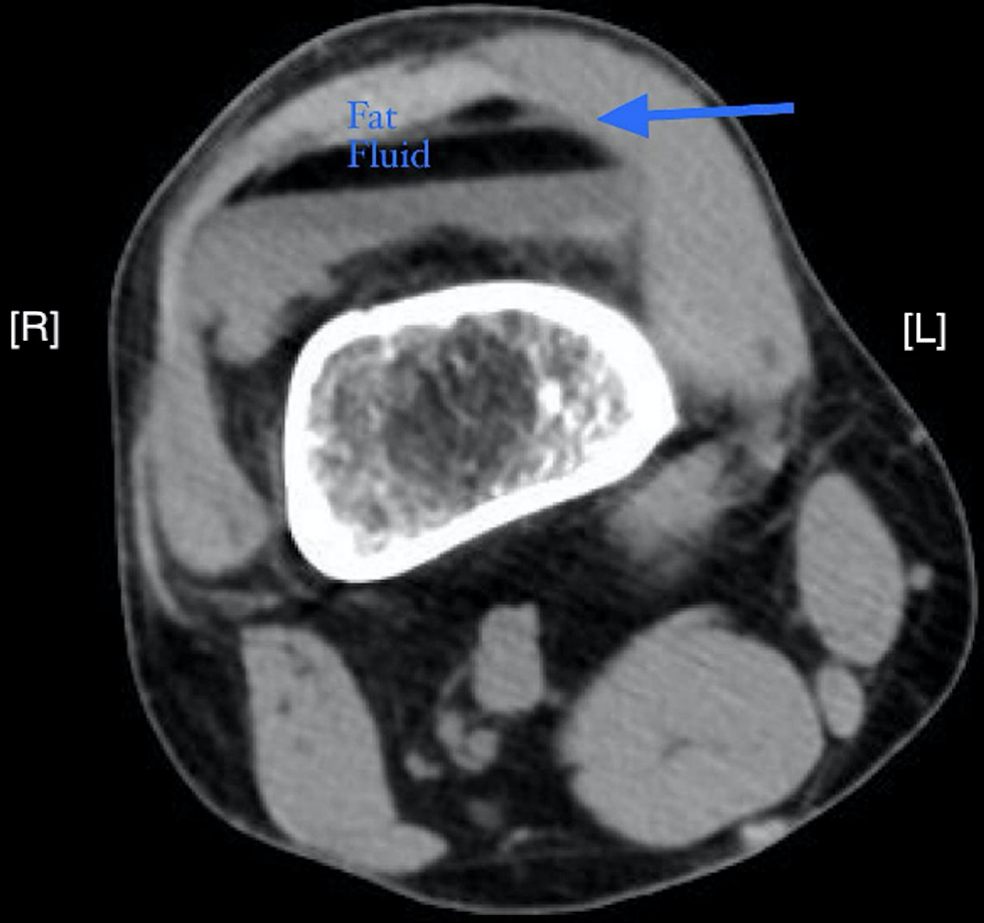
Axial CT image without contrast of the right lower extremity showed the fat-fluid level (blue arrow) indicative of lipohemarthrosis. Along with moderate lipohemarthrosis, soft tissue edema was identified. CT also revealed evidence of complex comminuted depressed fracture of the proximal tibia, involving mainly the lateral plateau. There is also a fracture of the fibular head
